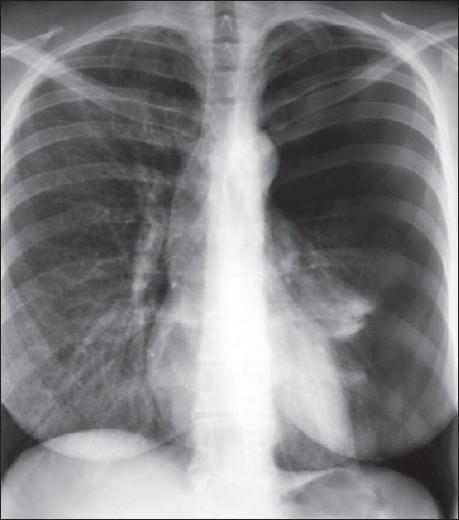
A frontal chest radiograph showing a large left side pneumothorax causing almost complete collapse of the left lung
